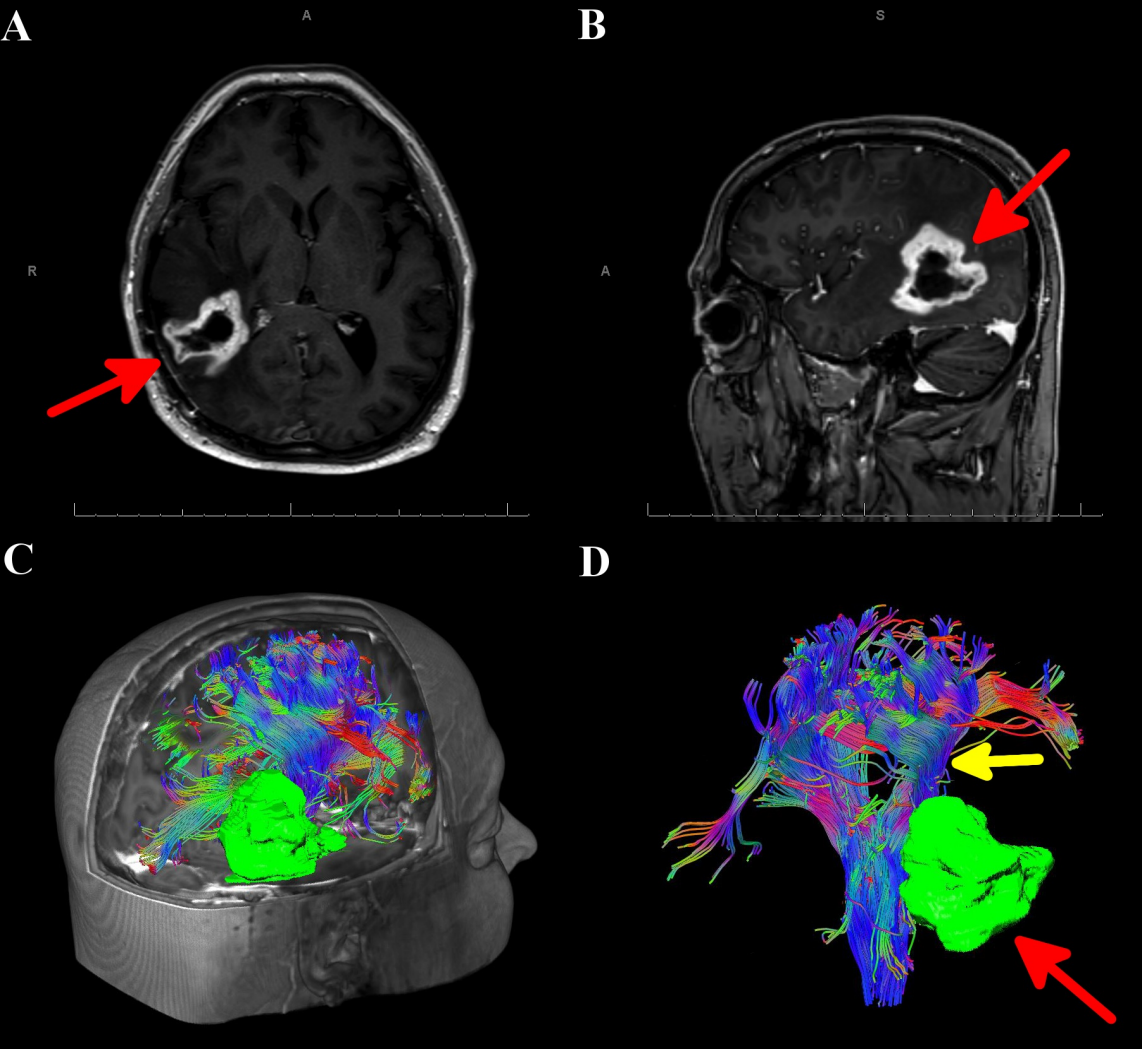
MRI of the brain and automated whole brain tractography(AWBT) for Case 2 A) Axial MRI of the brain with contrast showing a right-sided mass (denoted by red arrow). B) Sagittal MRI of the brain with contrast showing a right-sided mass (denoted by red arrow). C) AWBT image showing the tumor to be sitting deep and adjacent to the corticospinal tract. D) AWBT image showing the tumor (denoted by red arrow), sitting adjacent to the deep portions of the corticospinal tract (denoted by yellow arrow). AWBT: automated whole brain tractography; MRI: magnetic resonance imaging
